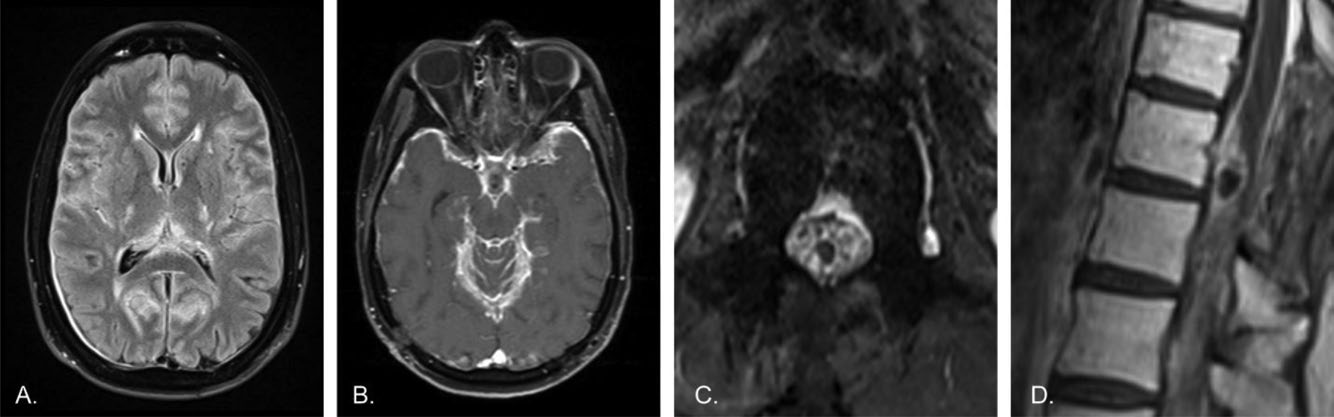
Worsening disease following radiation therapy and cessation of the MEK inhibitor trametinib due to side effects. A) Axial T2-FLAIR image of the brain shows worsened leptomeningeal hyperintensity. Dural thickening over the right occipital lobe is likely related to a craniotomy (not shown). B) Axial post-contrast T1 image of the brain demonstrates much thicker diffuse leptomeningeal enhancement. C) Axial post-contrast T1 image of the lumbar spine shows thick, diffuse enhancement of the cauda equina, now clearly outlining the conus as a dark circle in the center of the canal. D) Sagittal post-contrast T1 image of the lower thoracic spine demonstrates much thicker enhancement of the cord surface, and continued enhancement of the dorsal mass, which also shows biopsy changes. Note the medullary hyperintensity, consistent with history of radiation therapy.
